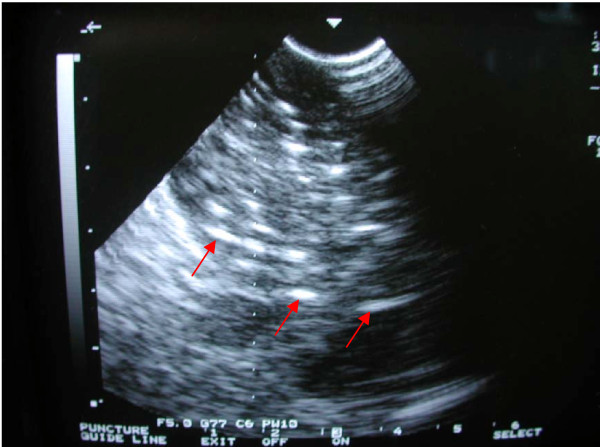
Intraoperative ultrasound scan showing the distribution of implanted seeds in the tumor.
